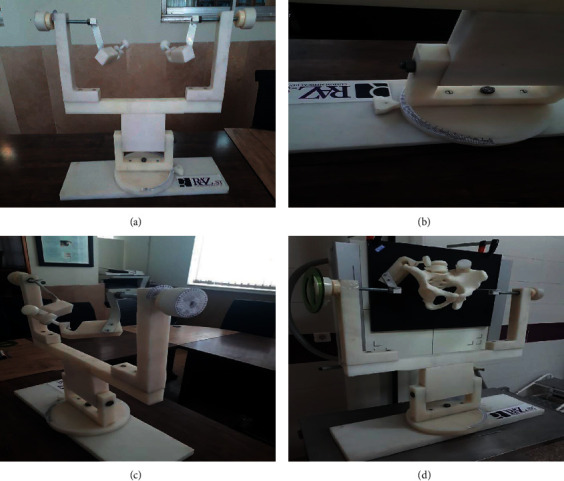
(a) Pelvic holder. (b) The degree of rotation maintenance. (c) Tilt degree maintenance. (d) Utilizing the pelvic holder for roentgenography of the pelvic model.
